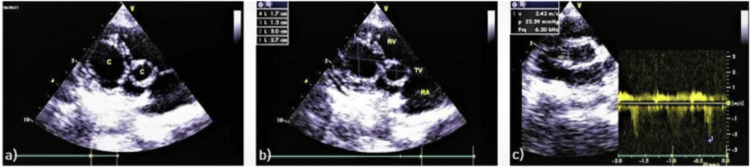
Transthoracic echocardiography images showing (a) two cystic lesions in the right ventricle, (b) dimensions of the two cysts and, (c) mild preoperative tricuspid regurgitation (right ventricular pressure 23 mmHg). Image and caption reproduced from Aljaber et al., 2020 [15] under the terms of the Attribution-NonCommercial-NoDerivatives 4.0 International license (https://creativecommons.org/licenses/by-nc-nd/4.0/).
